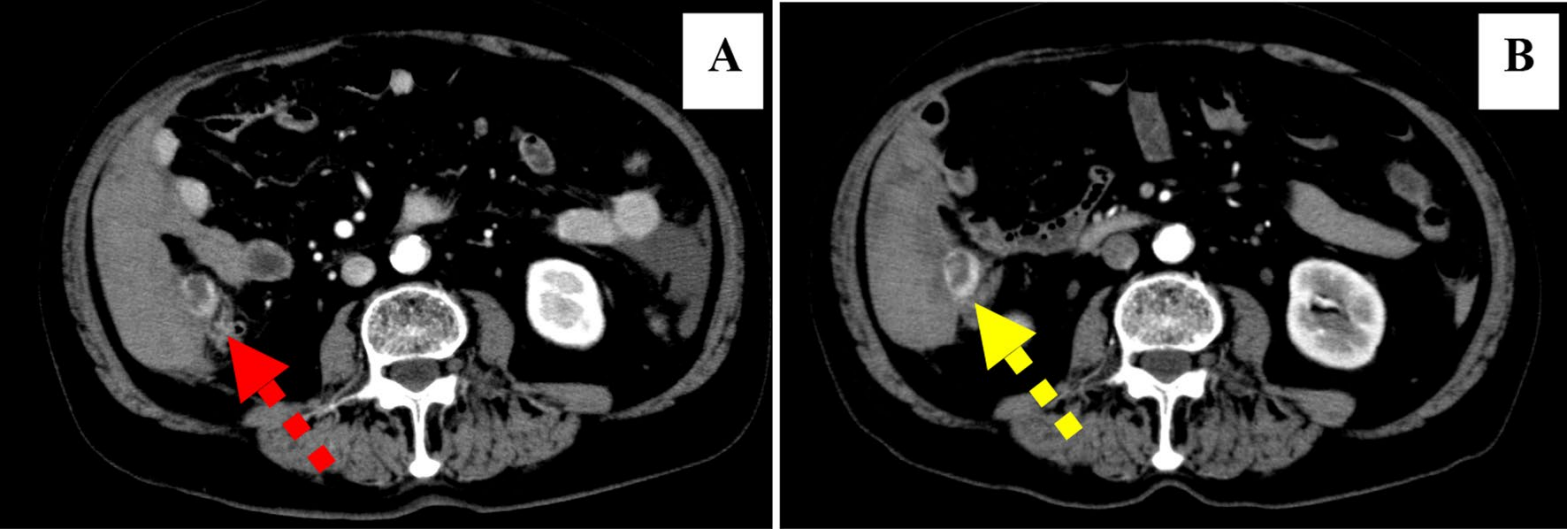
Preoperative contrast-enhanced CT. A CT image of the patient when he first came to the hospital with abdominal pain. B Preoperative CT image when the abdominal pain recurred 1 week after (A). A ring-shaped contrast area (arrows) between the ascending colon and the retroperitoneal hematoma. The anemia had worsened, but the size of the hematoma had not changed significantly
